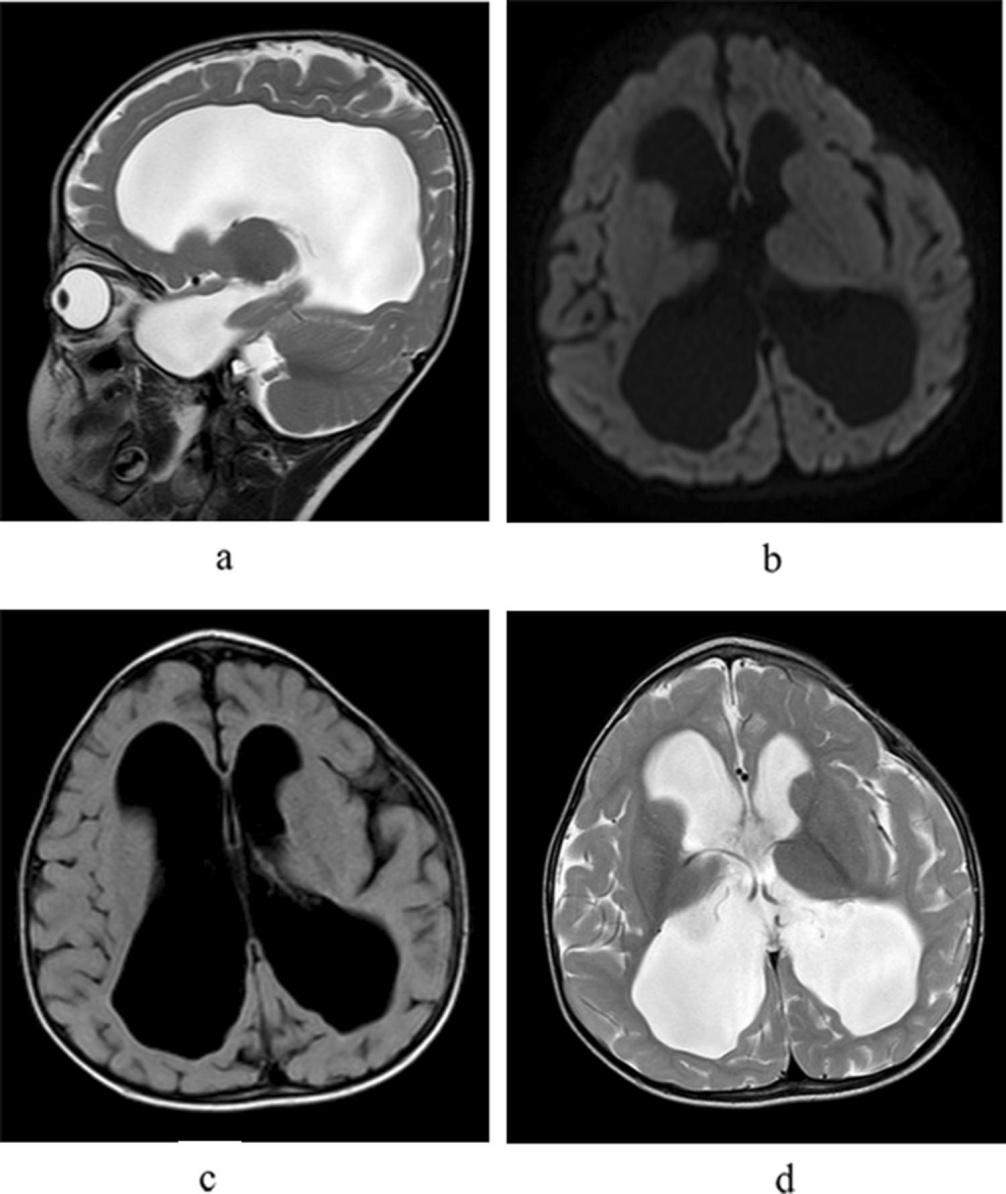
(a–d) Patient no. 30. MRI showing severe ventricular dilation and corpus callosal thinning (a, sagittal T2WI).
